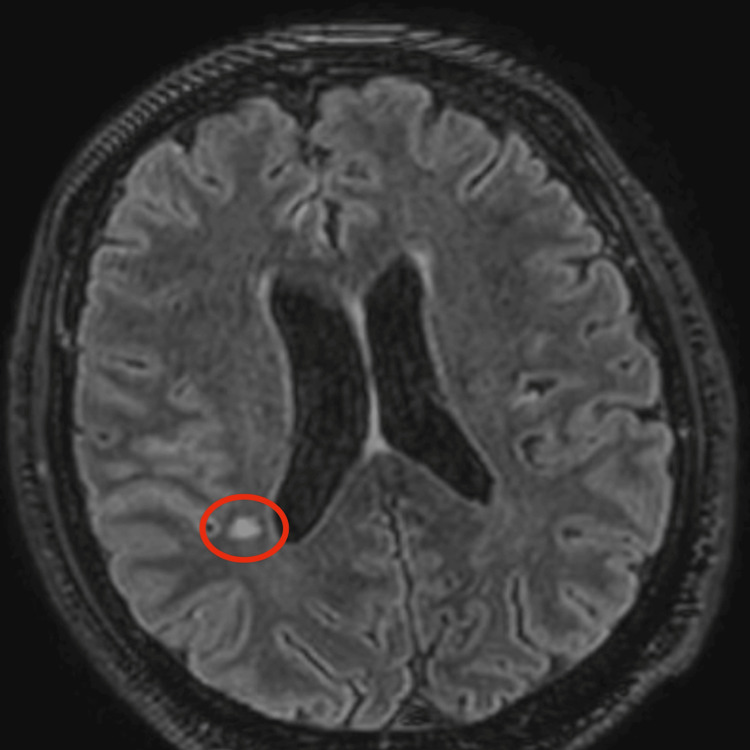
Axial post-contrast FLAIR MRI demonstrating focal leptomeningeal enhancement in the right posterior frontal region, adjacent to the insular cortex, with an adjacent cortical-subcortical hyperintense lesion consistent with subacute ischemic infarction (red circle highlights the cortical-subcortical hyperintense lesion). FLAIR: fluid-attenuated inversion recovery; MRI: magnetic resonance imaging
